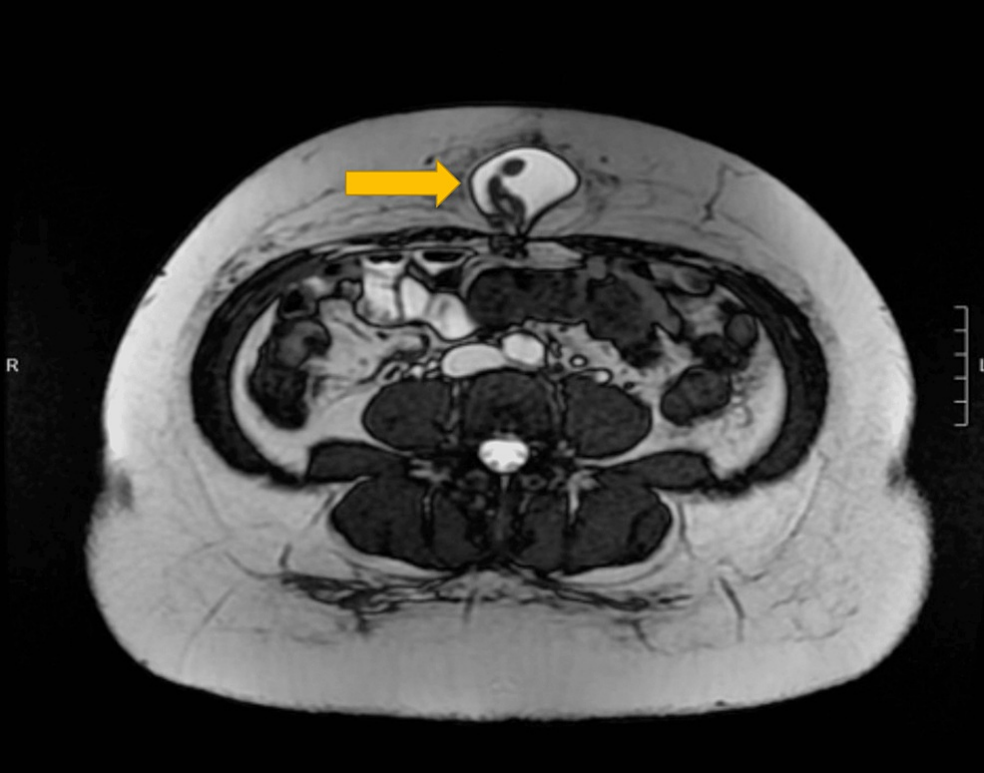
Preoperative MRI MRI with arrow pointing to ventral ligament hernia. The falciform ligament was diagnosed intraoperatively.
